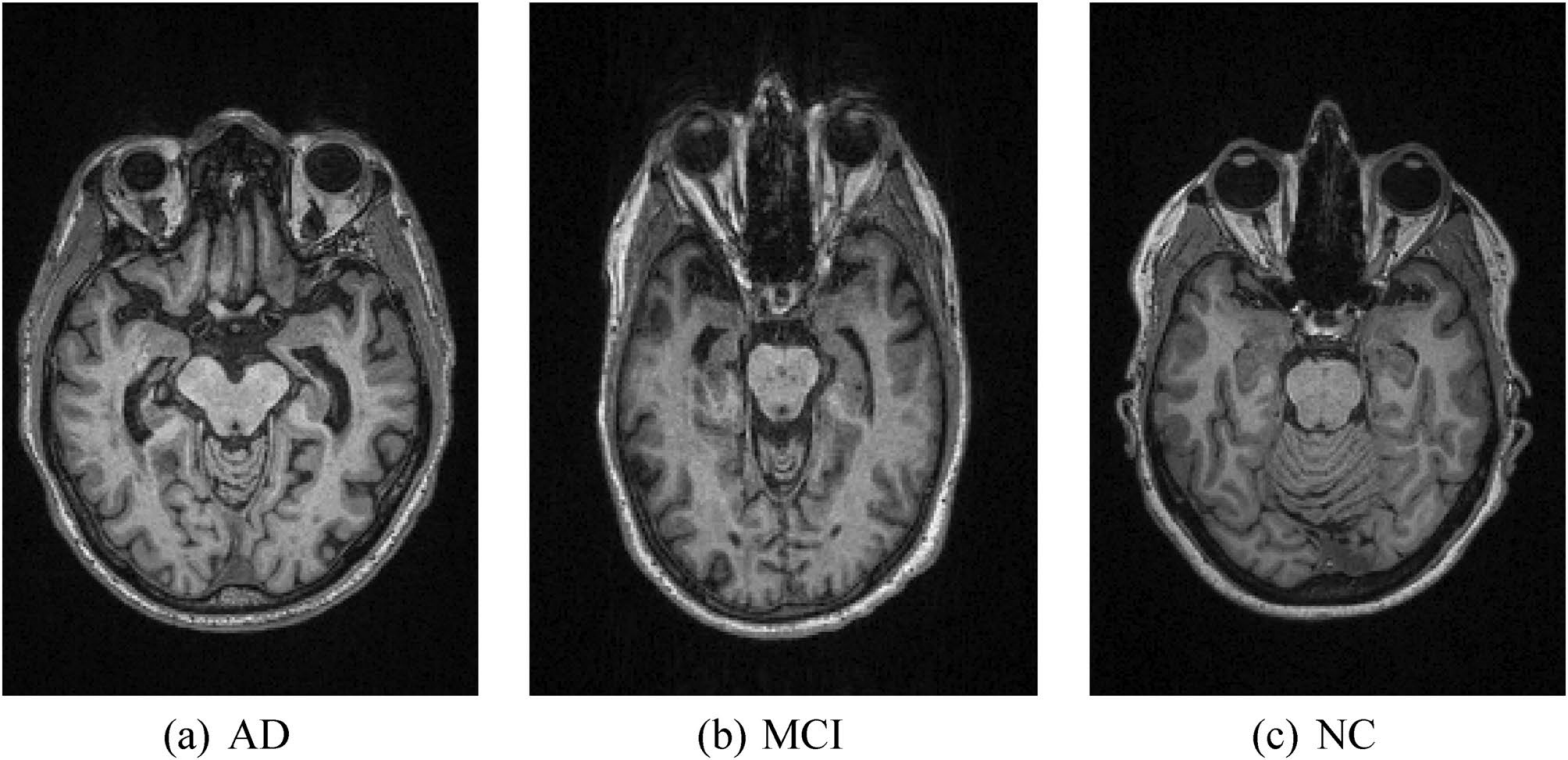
Examples of MR images from OASIS in different classes of AD.
